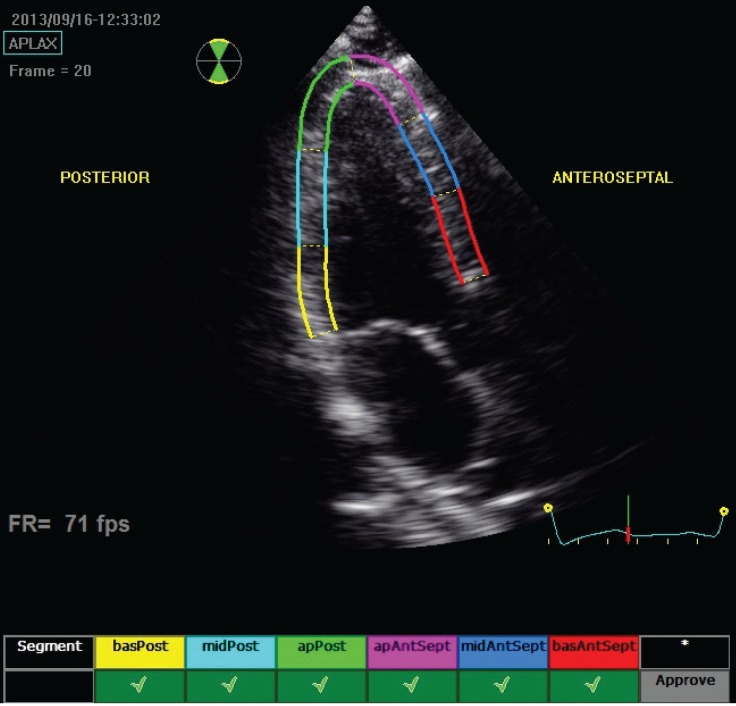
Echocardiograph shows segmental analysis of the left ventricle after 2D speckle tracking from the apical long-axis view.
